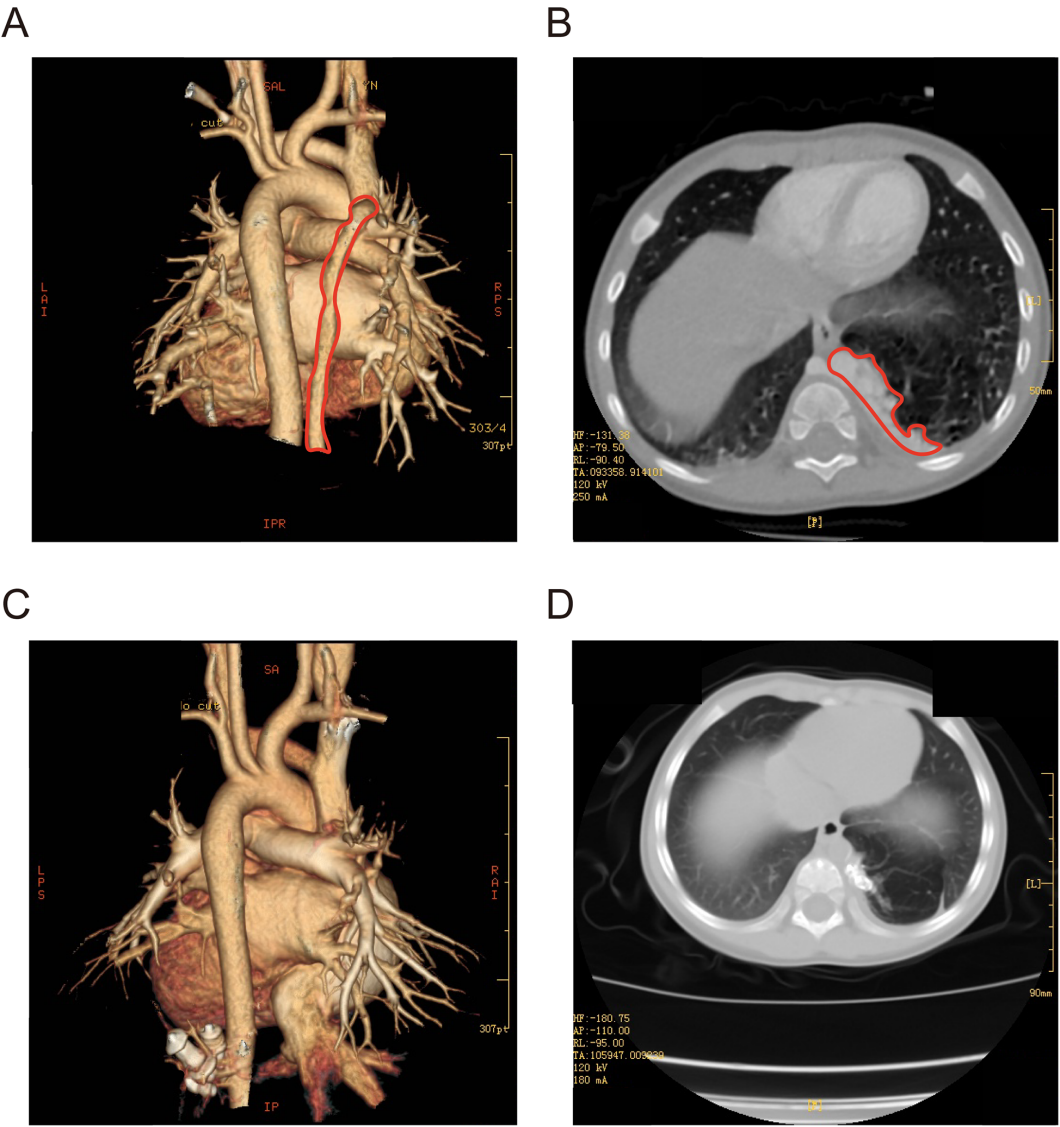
(A,B) Three-dimensional CT of the chest before surgery (marked in red for PS). (C,D) Three-dimensional CT of the chest 12 months after surgery.
